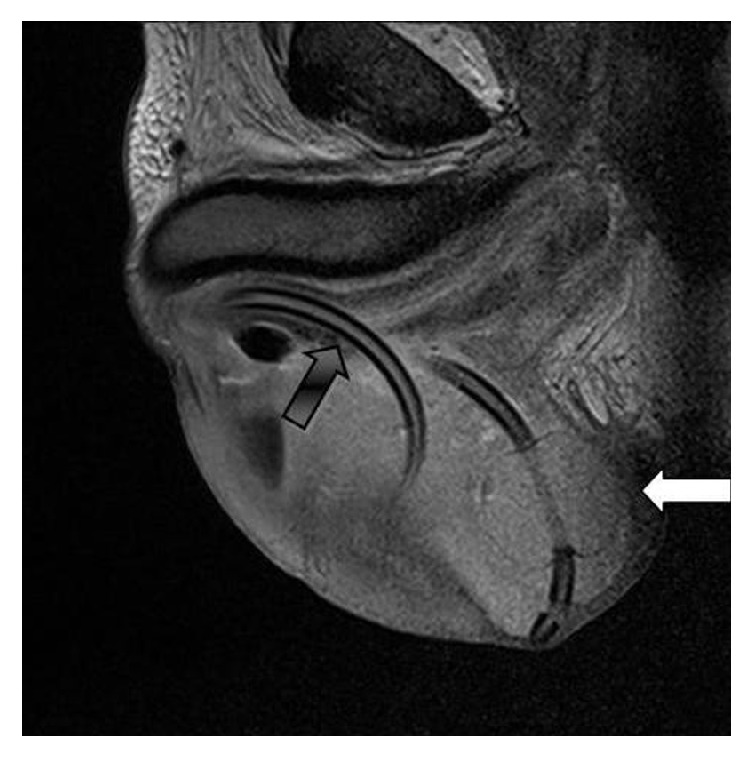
Scrotal MRI (sagittal): urethroscrotal fistula (black arrow) with catheter rolled in the scrotal sac, balloon (white arrow).
